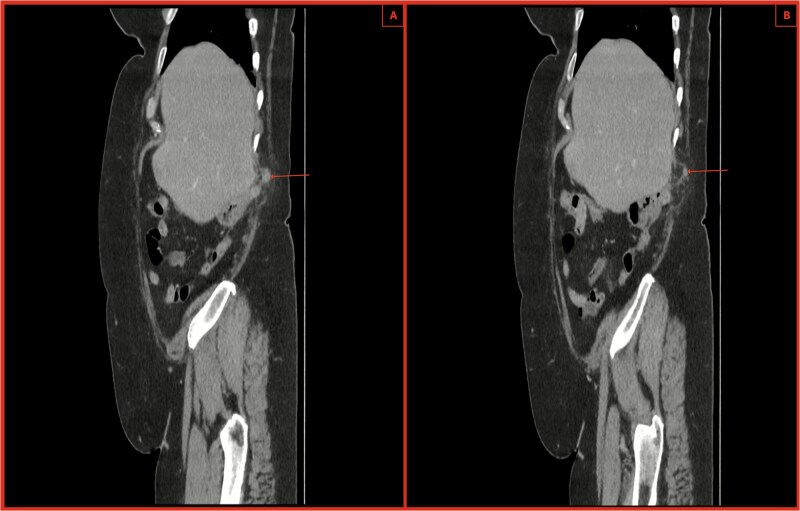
(A, B) CT sagittal sections with notable posterior abdominal wall hernial defect and appendix within hernia highlighted by arrows. There is also notable inflammatory fat stranding, with no evidence of perforation.
